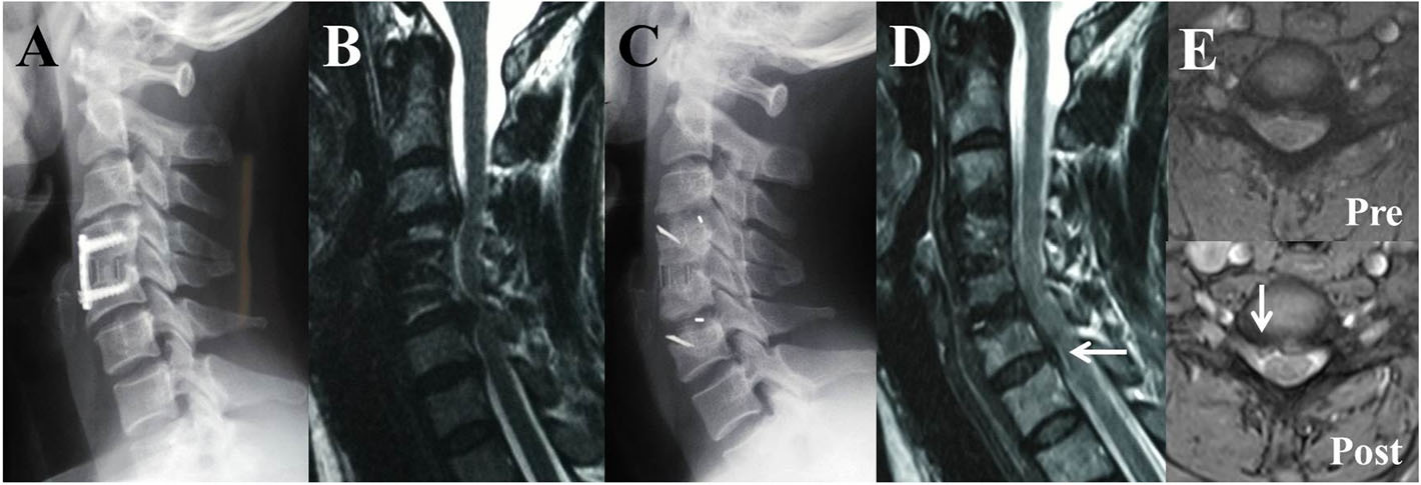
A 41-year-old woman developed two-level ASD with radicular symptoms at the 5th year after the primary surgery and underwent ACDF with two Zero-P cages as a revision surgery. a Lateral radiograph after the primary surgery showing that ACDF was performed at C4–C5. b MRI before revision surgery showing new degenerative changes at C3–C4 and C5–C6 causing stubborn upper limb pain and numbness. c Lateral radiograph after revision surgery showing that ACDF was performed at both the cranial and caudal levels. d MRI at 2 years postoperatively showing adequate decompression at the C3–C6 levels but new posterior disc herniation at C6–C7 (arrows). e Axial MRI at C6–C7 before and after the revision surgery showing aggravated disc herniation (arrows). The patient suffered from intermittent radiating pain in the right arm that was remediated by conservative treatment.
